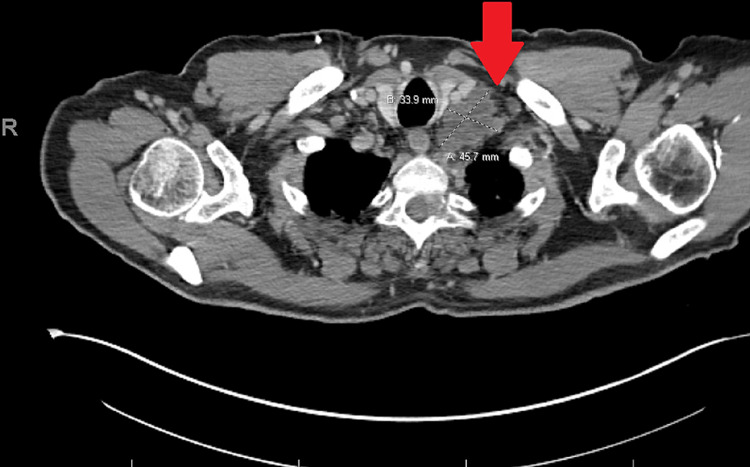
CT image of the pelvis CT image of the pelvis showing conglomeration of pathologic adenopathy and left supraclavicular region (Virchow’s node) measuring 4.6 x 3.4 cm. Additional left supraclavicular node measures 2.4 x 1.6 cm.
